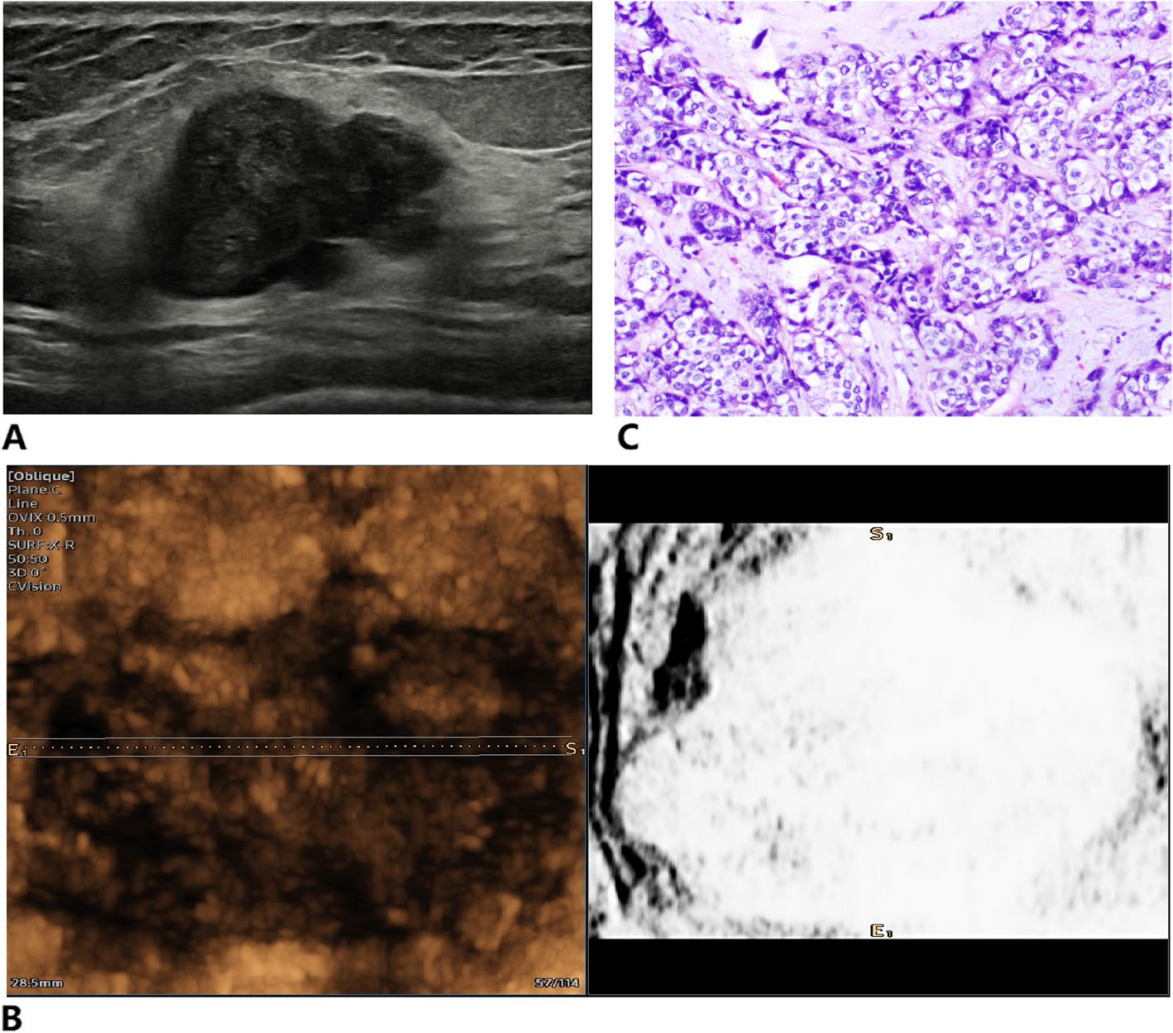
Infiltrating ductal carcinoma of the right breast. A Two-dimensional ultrasound image was classified the breast mass as BI-RADS 4A. B Inversion imaging image showing a white mass with less than three NTRs, and the point of inversion was 5. C Pathological section showing that the mass was an invasive ductal carcinoma
